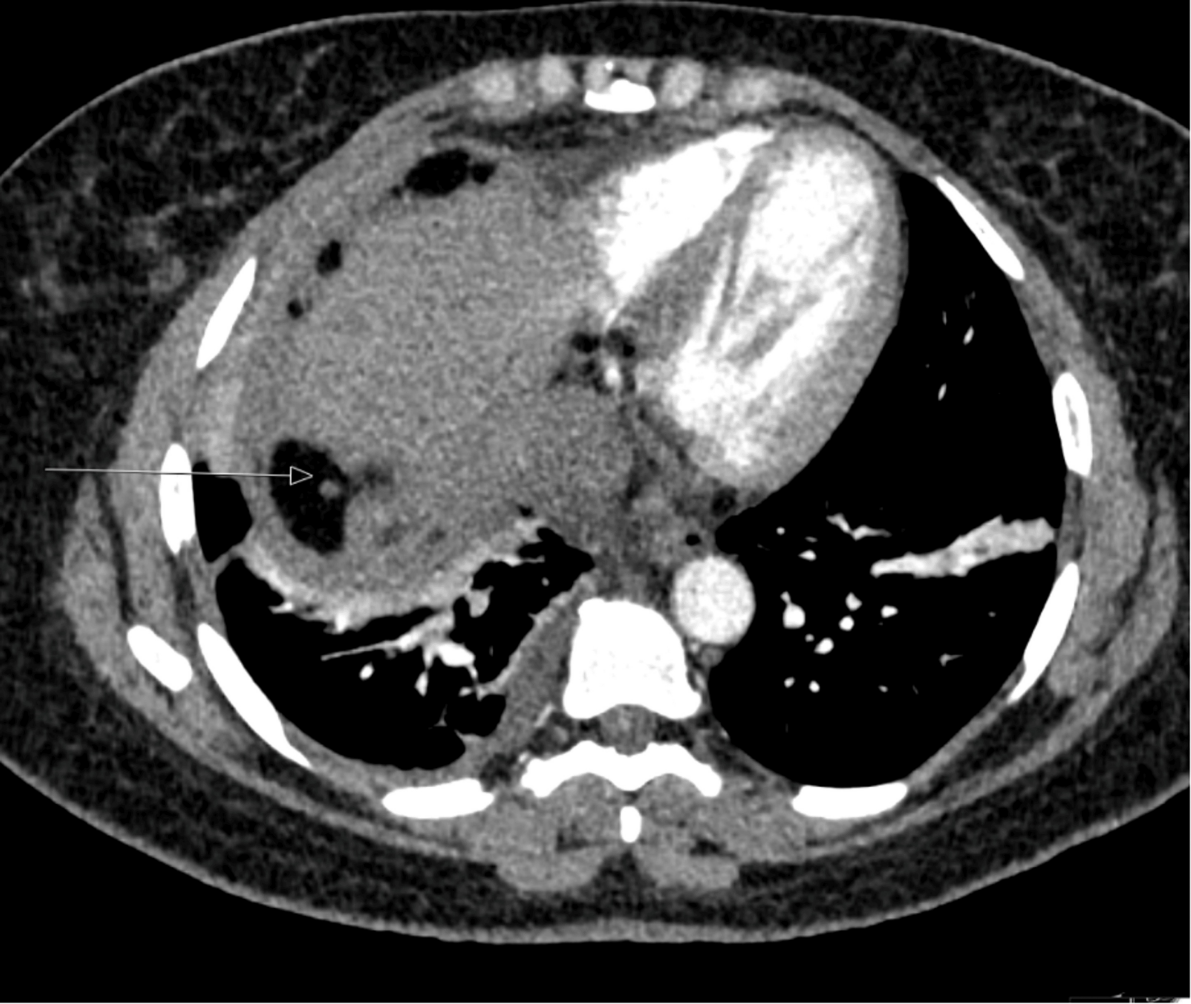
CTPA Showing Right-Sided Consolidation Axial CTPA image showing no pulmonary embolism but right lower-lobe consolidation and adjacent pleural thickening, consistent with inflammatory change and with a significant fat lobule under the diaphragm (arrow). CTPA: computed tomography pulmonary angiogram.
